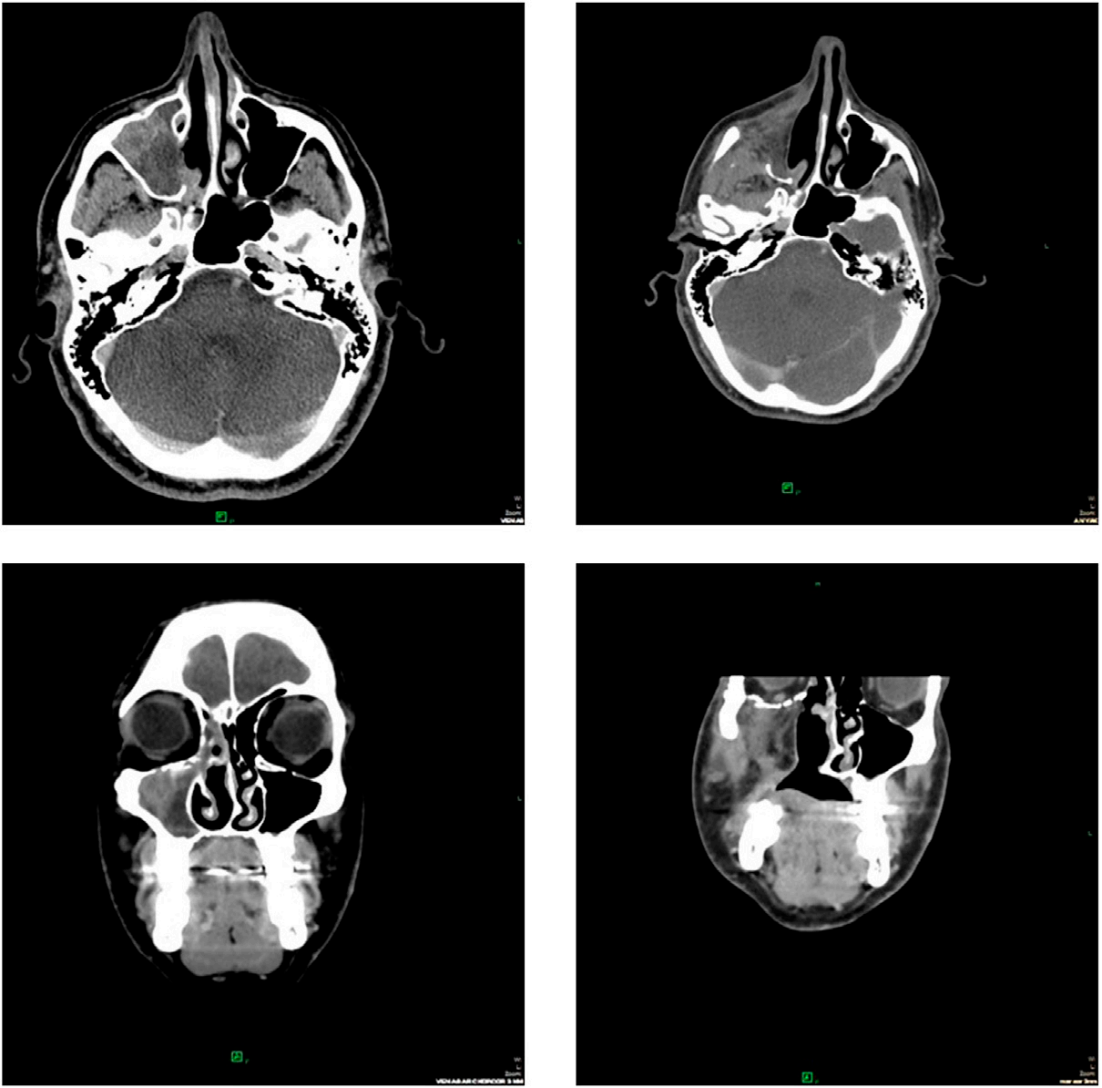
CT scans after 6 months of the irradiation showed partial response of the primary melanoma in the nasal cavity. The patient received chemotherapy as systemic treatment.
